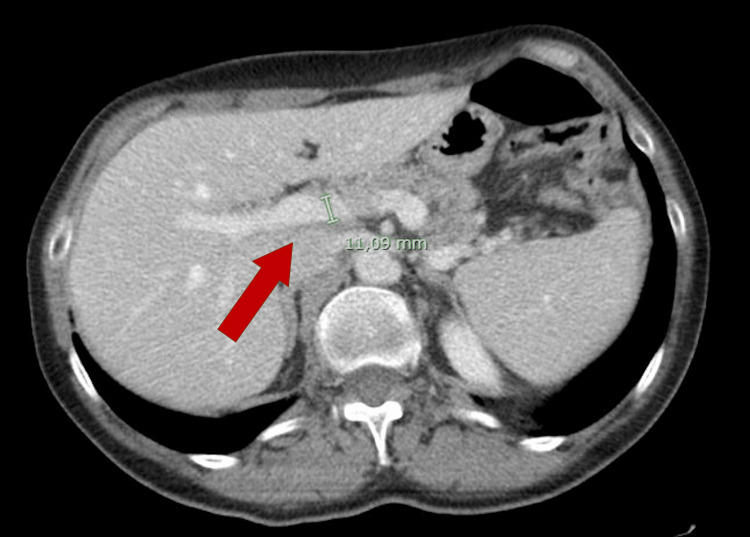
CT scan before T-DM1 treatment was started, showing normal portal vein diameter (red arrow).
